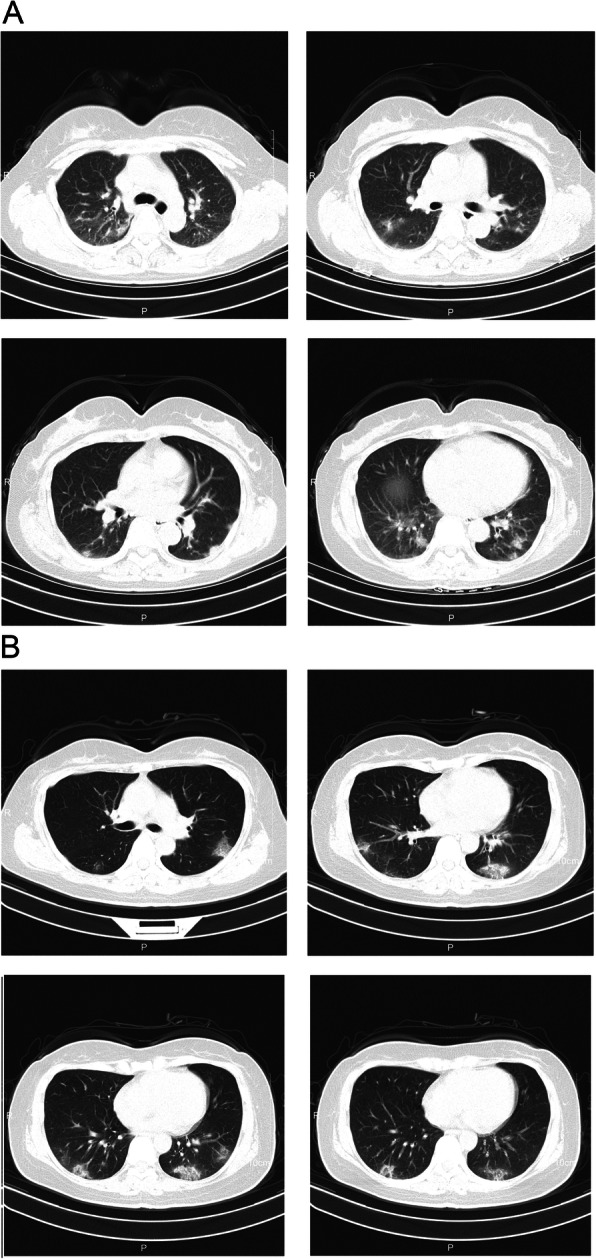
Transverse chest computed tomography (CT) images. a, The CT images from a woman (age range: 55–59 years old) at admission show bilateral ground-glass opacities with blurred borders; b, The CT images from a woman (age range: 45–49 years old) at admission shows multiple patchy ground-glass shadows in both lungs, predominately in the lower lobes, with signs of anti-halo
